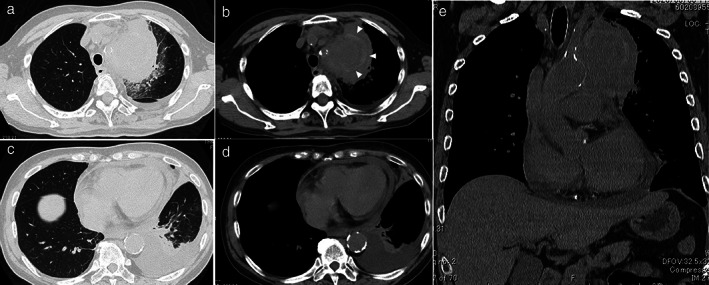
Chest computed tomography (CT) on admission. (a, c) Lung windows. (b, d, e) Soft‐tissue windows. Chest CT shows a tumor shadow in the upper lobe of the left lung, pleural effusion, and pericardial effusion. A region of ground‐glass attenuation is apparent around the tumor. A linear region of high attenuation (arrowheads) is apparent inside the tumor.
